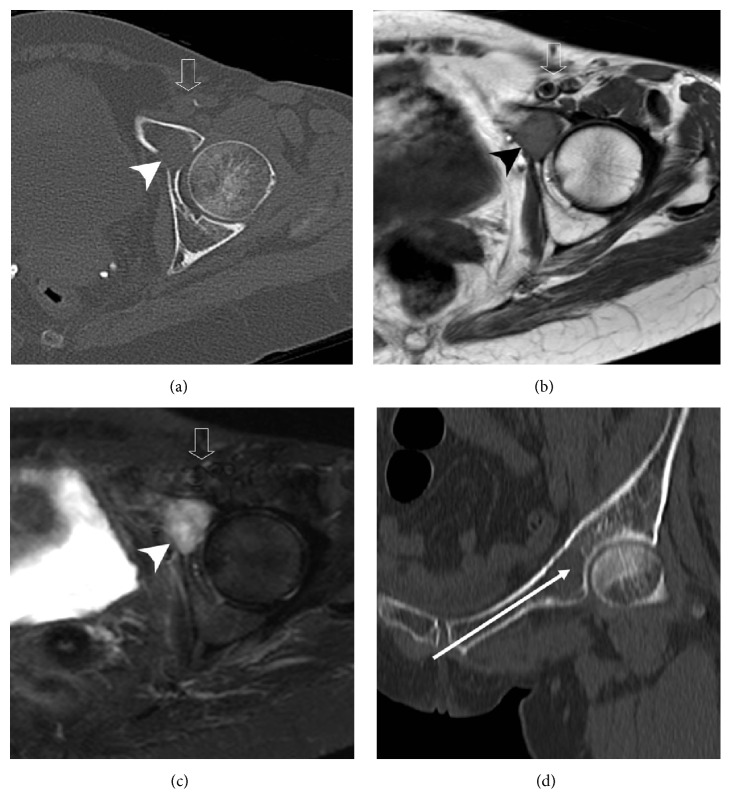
60-year-old-female with metastatic lung cancer complaining of left hip pain. (a) Nonenhanced axial CT image demonstrates left anterior acetabular osteolytic lesion (arrowhead) with anterior cortical thinning and posterior cortical breach. (b) and (c) The acetabular lesion (arrowhead) shows low signal intensity on axial T1W image (b) and high signal intensity on axial STIR image (c). Note its close relationship with the femoral neurovascular structures precluding a direct anterior approach (open arrow). (d) Coronal oblique reformatted CT image shows the needle path from the skin entry to the target lesion (long arrow).
